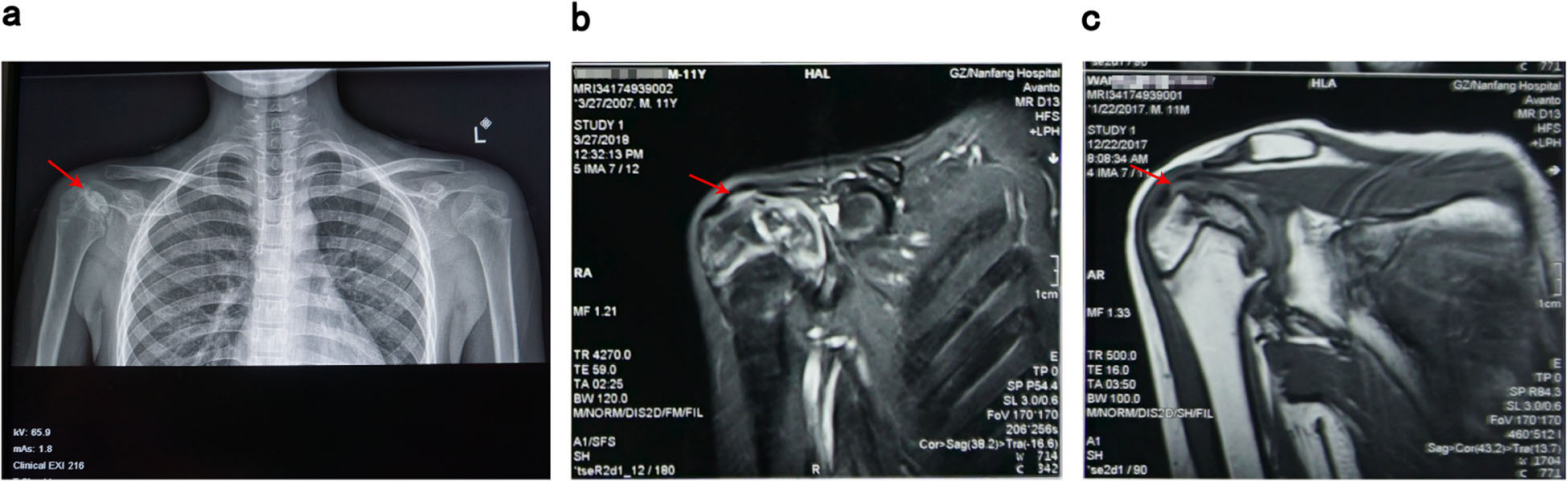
X-ray imaging (a) and magnetic resonance imaging (b, c) results of right shoulder joint (The arrows point out pathological structures in examinations, indicating necrosis of the right shoulder osteoepiphysis)
